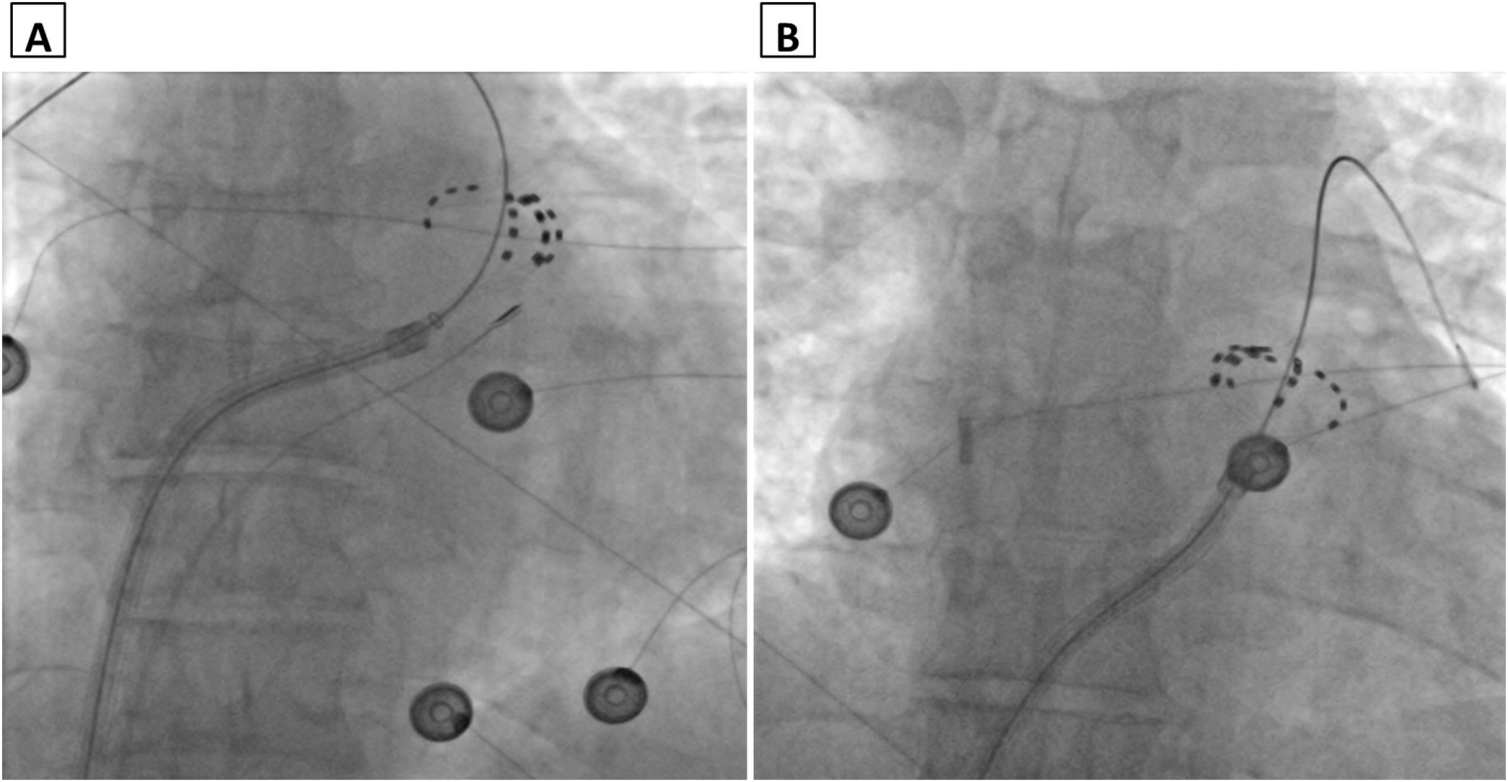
Fluoroscopy image (antero-posterior view) of the pentaspline catheter in “basket” configuration with intracardiac echocardiography catheter in the right ventricular outflow tract (A) and right atrium (B).
